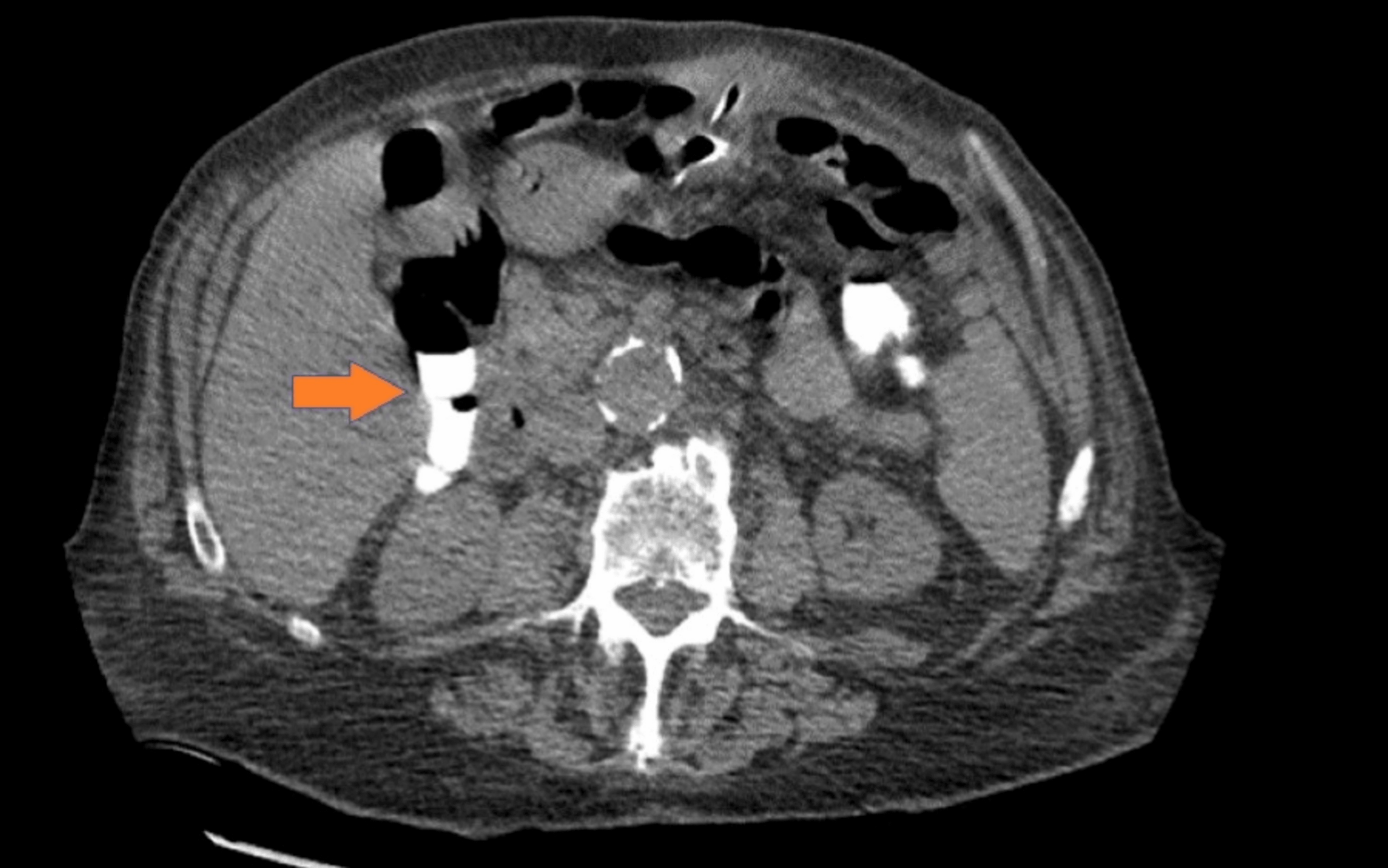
CT scan of abdomen and pelvis with contrast through the PEG tube showing contrast in the colon. PEG: Percutaneous endoscopic gastrostomy
